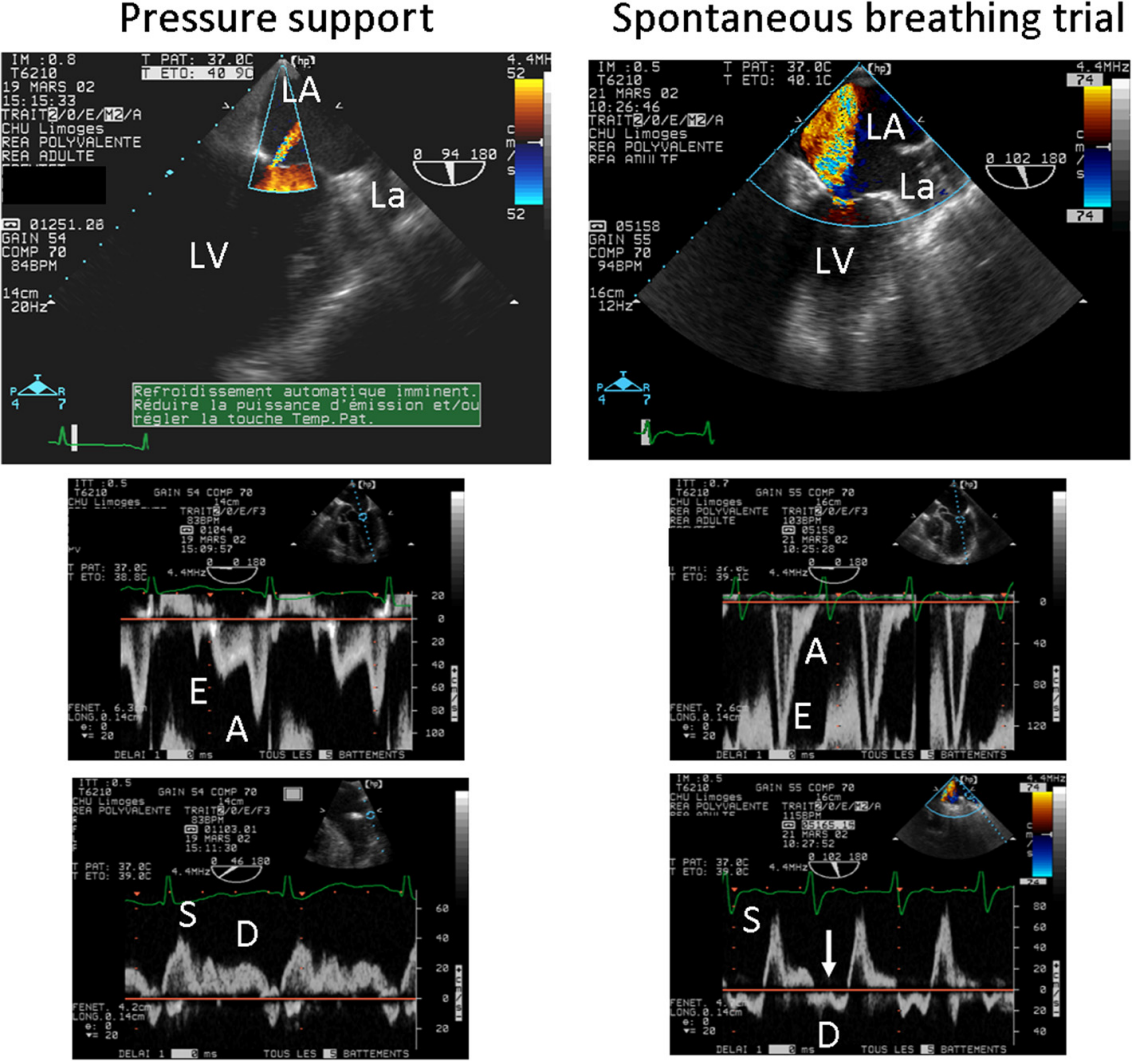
Transesophageal echocardiographic hemodynamic monitoring in a patient with a known ischemic cardiomyopathy who failed ventilator weaning. Under pressure support, a trivial mitral regurgitation was disclosed by color Doppler mapping in the two-chamber view (*upper left*), and left cardiac filling pressures were low, as reflected by an inverted mitral E/A Doppler pattern (*middle left*) and a predominant pulmonary vein S wave (*lower left*). During the spontaneous breathing trial, a severe mitral regurgitation occurred (*upper right*), mitral Doppler pattern was restrictive (*middle right*), and pulmonary vein Doppler disclosed a reversed D wave consistent with massive mitral insufficiency (*lower right*, *arrow*). The acute mitral regurgitation was attributed to a papillary muscle dysfunction secondary to a transient myocardial ischemic event. *LA* left atrium, *La* left auricle, *LV* left ventricle. Adapted from [7]
